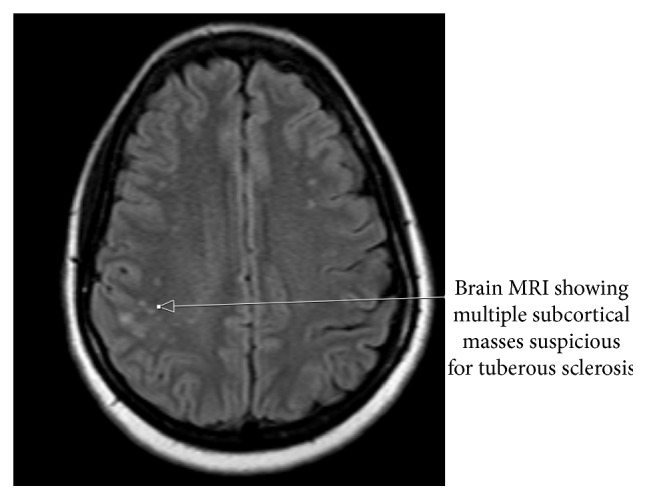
Brain MRI, showing subcortical masses.
